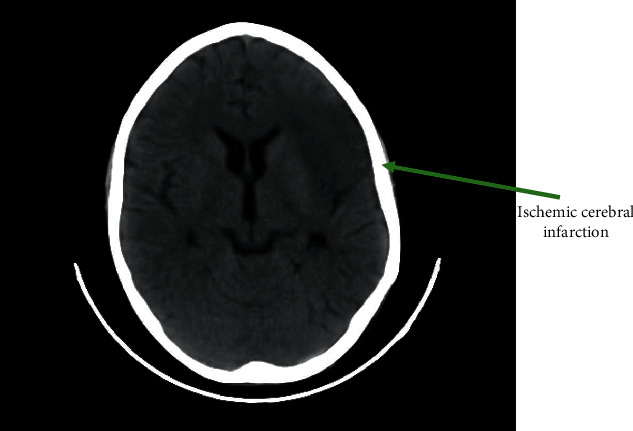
A cerebral CT scan revealing a hypodense lesion on the left frontal lobe indicating a recent ischemic cerebral infarction.
